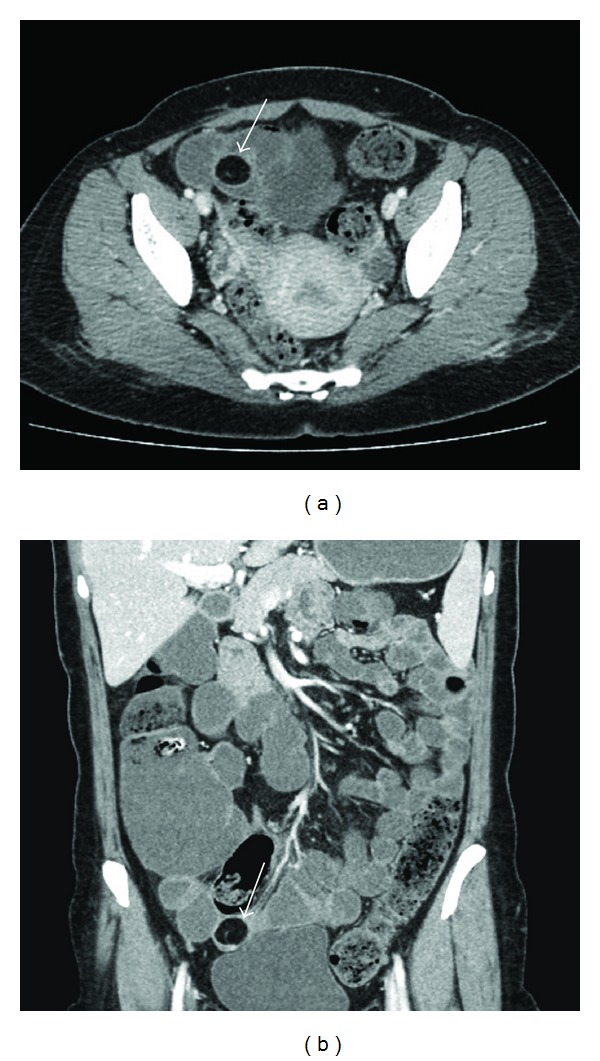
(a) Axial and (b) coronal plan contrast-enhanced CT scans demonstrate a well-circumscribed, intraluminal hypodense 25 × 20 mm mass with fat attenuation (−105 HU) in the terminal ileum (arrow). Any obvious sign of obstruction is not present on this CT.
